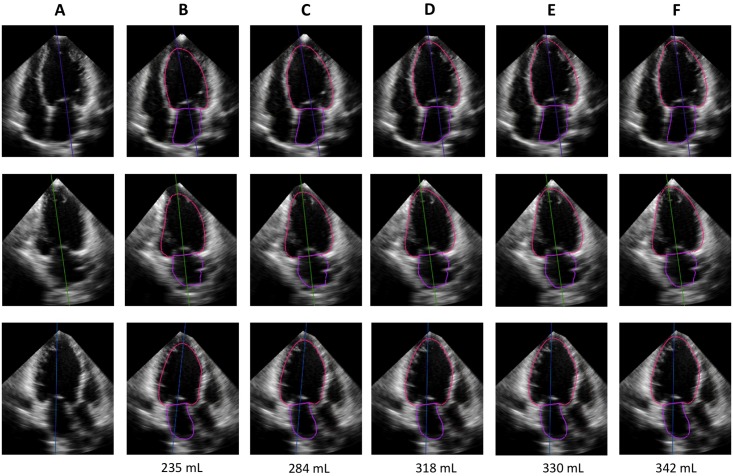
Left ventricular end-diastolic endocardial border tracing line used by different LV endocardial border threshold in a representative case. Upper, middle, and lower panels show apical 4-chamber, 2-chamber, and long-axis views extracted from 3D dataset. A, no border line; B, border threshold of 0; C, border threshold of 50; D, border threshold of 80; E, border threshold of 90; F, border threshold of 100.
